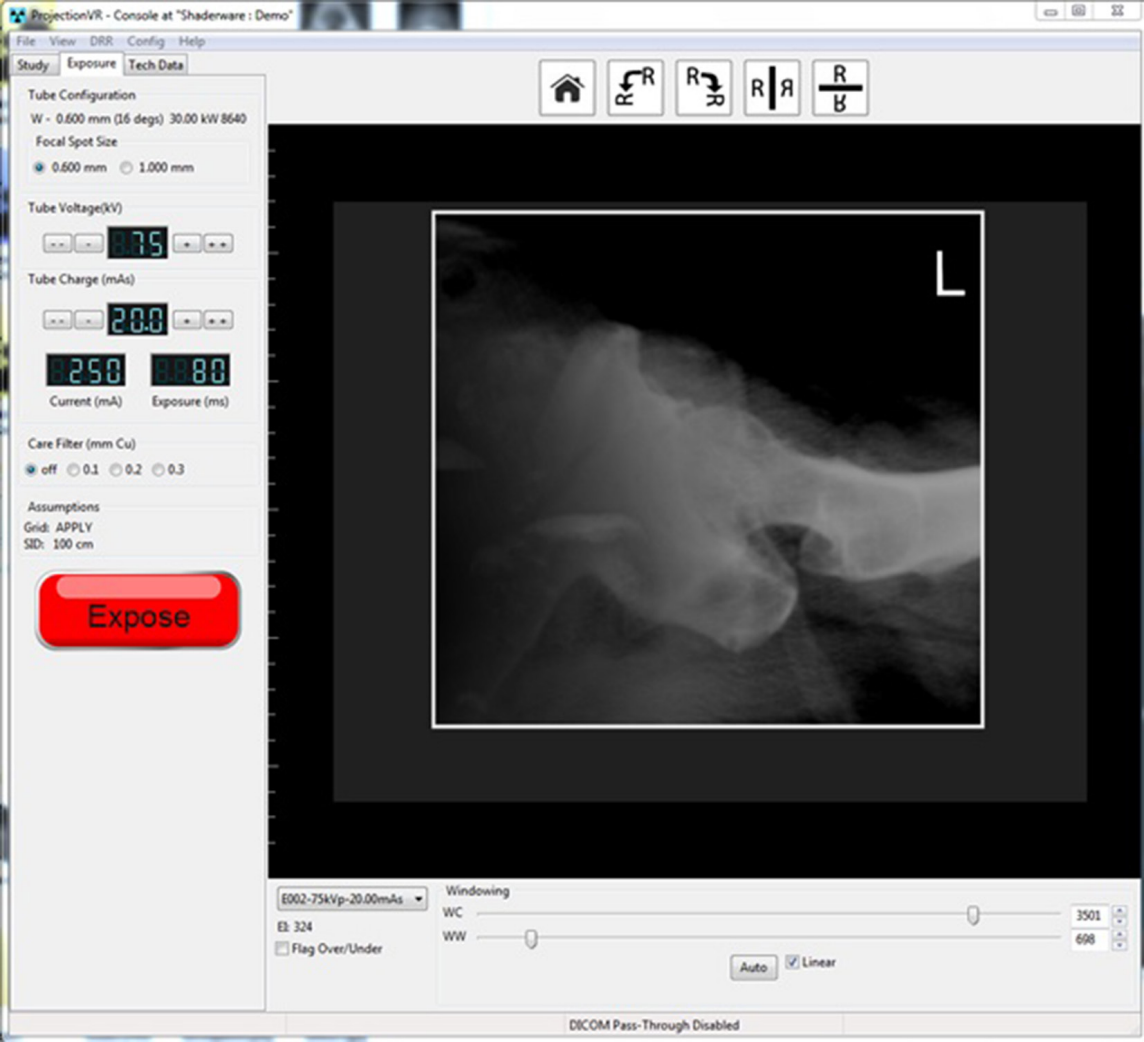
ProjectionVR^TM^ (v. 5.0) X-ray console displaying digitally reconstructed radiograph (DRR).
